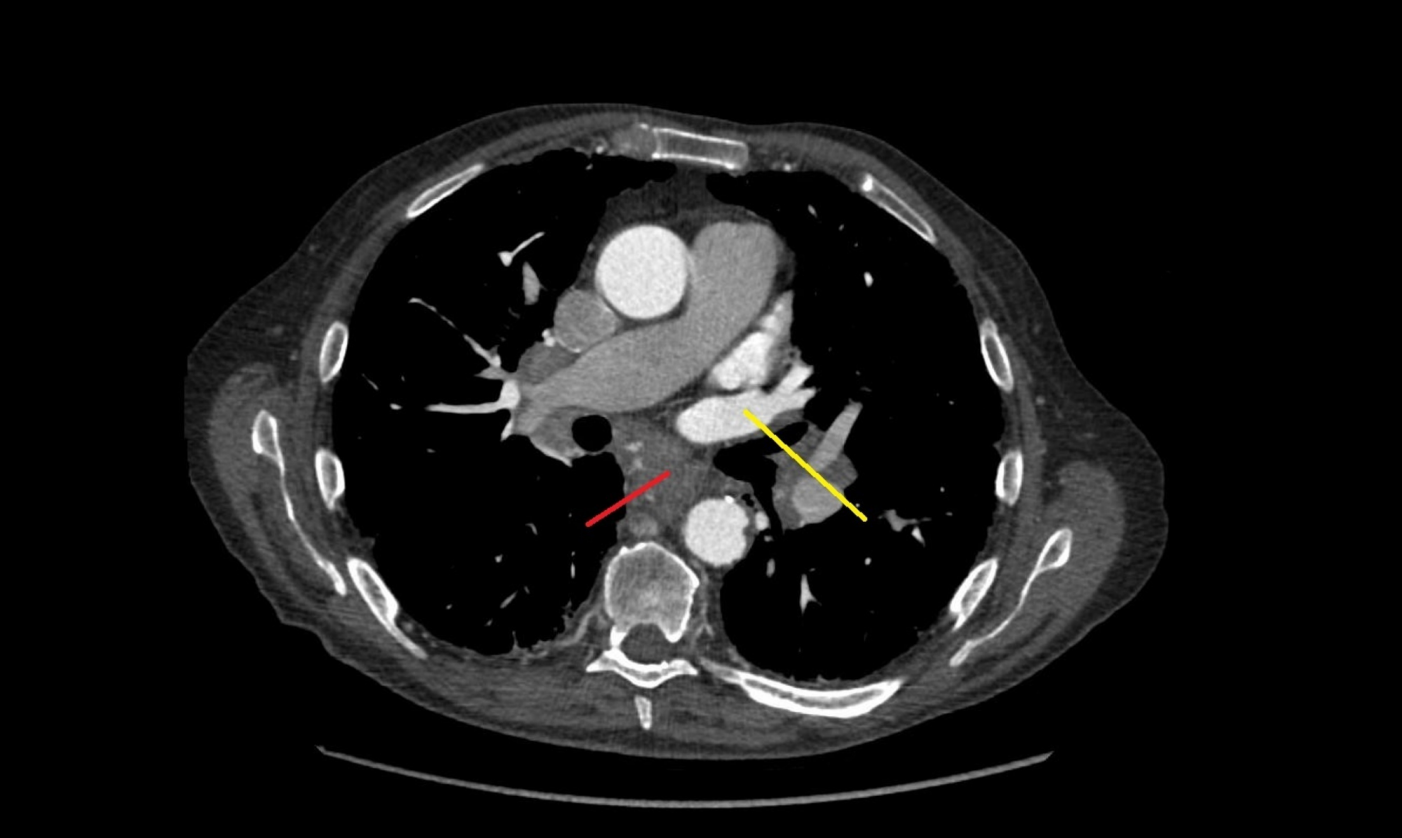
Computed tomography (CT) of the thorax with contrast showing left upper lobe mass with mediastinal involvement (red pointer) and encasement of left pulmonary vein (yellow pointer)
